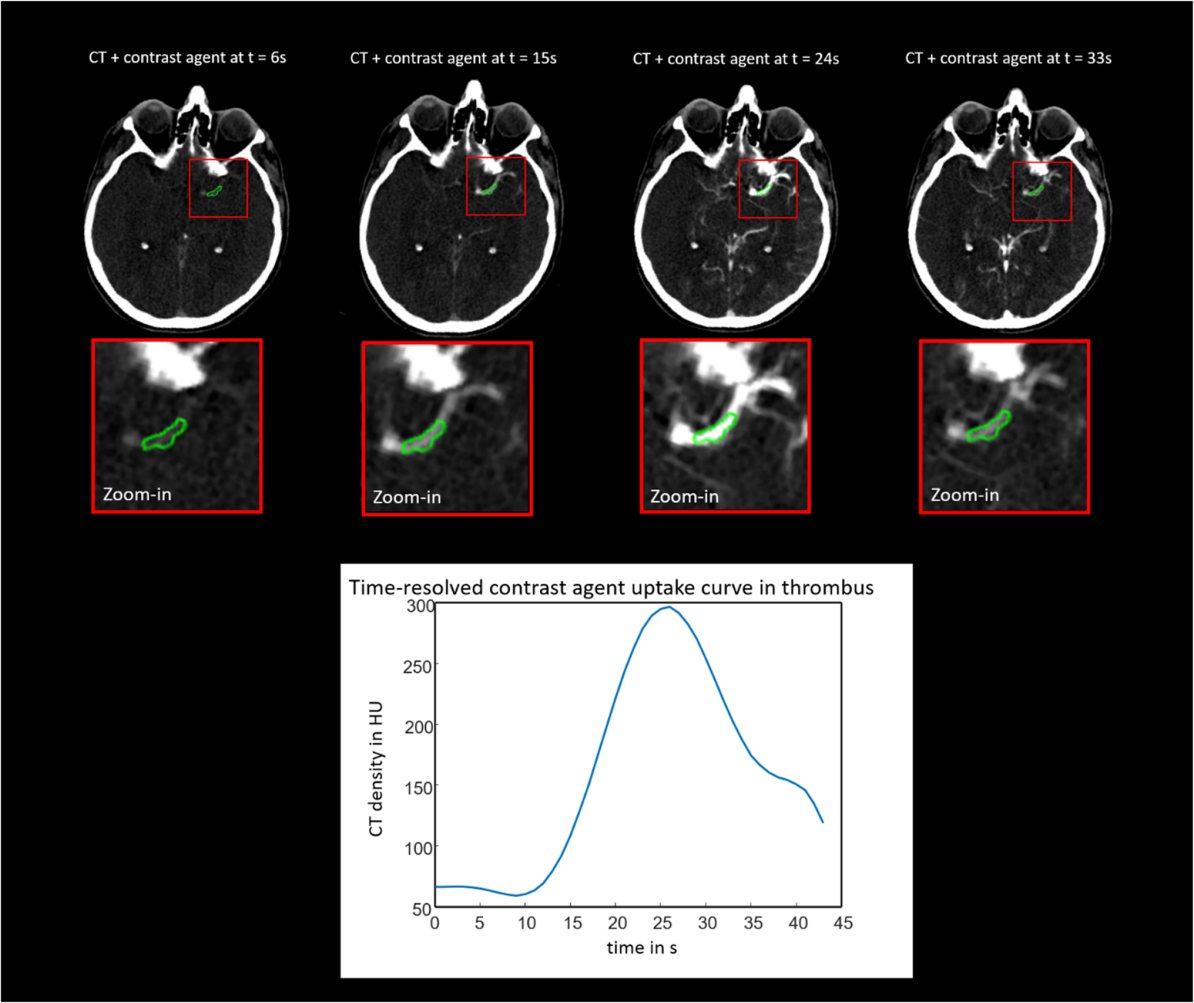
Time resolved contrast agent uptake curve (CAU) in a thrombus volume determined using the time-resolved imaging series of a CT perfusion scan. TAI_standard_ characterizes this contrast agent uptake curve with only two imaging time points, which can lead to substantial mischaracterization of thrombi through a signal processing phenomenon known in the literature as “under-sampling”. CTP parameters: 80 kV tube voltage, 3.0 mm slice thickness, 512 × 512 image size with 0.4 × 0.4 mm in-plane resolution, 30 phases with 1.5 s increments, 45 s total scan time
